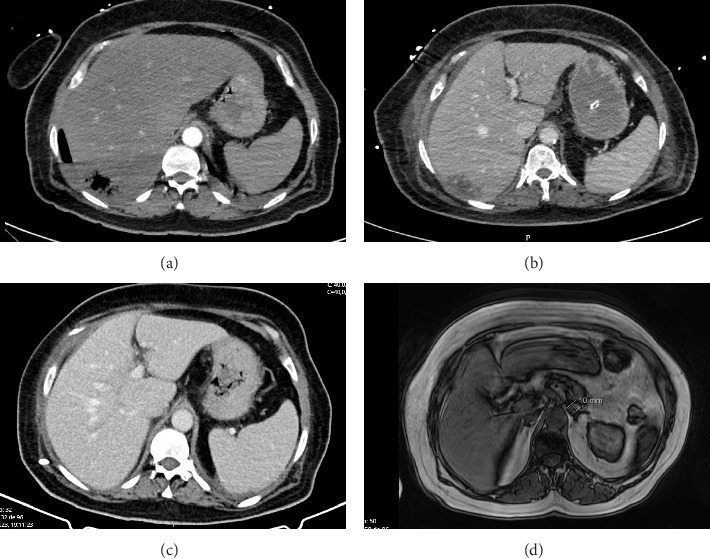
Imaging evolution of the hepatic abscess. (a) (Admission): abdominal computed tomography (CT) showing a VII segment hepatic abscess; (b) (3 days after admission): CT showing the hepatic abscess 54 × 30 mm with a pigtail drain; increment of the liquid component of the abscess; (c) (one-month after admission): CT showing decrease of the VII segment hepatic abscess (37 × 17 mm), pigtail drain inside; and (d) (9-month follow-up after discharge): magnetic resonance imaging T1 showing hepatomegaly with signs of steatosis; complete resolution of the abscess previously viewed in segment VII.
